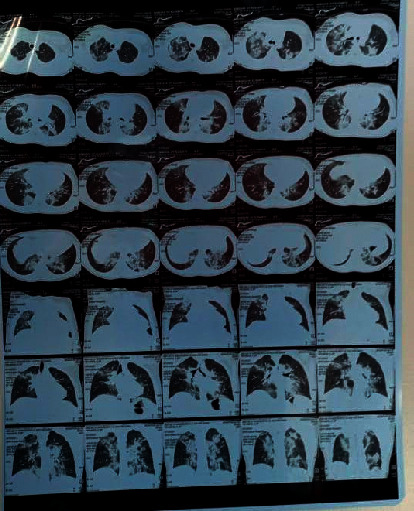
A film of the chest computerised tomography scan: features of multifocal peripheral ground glass opacification and consolidation in both lungs with basal and apical predominance. The CT severity score is 14/25 (moderate parenchymal involvement).
